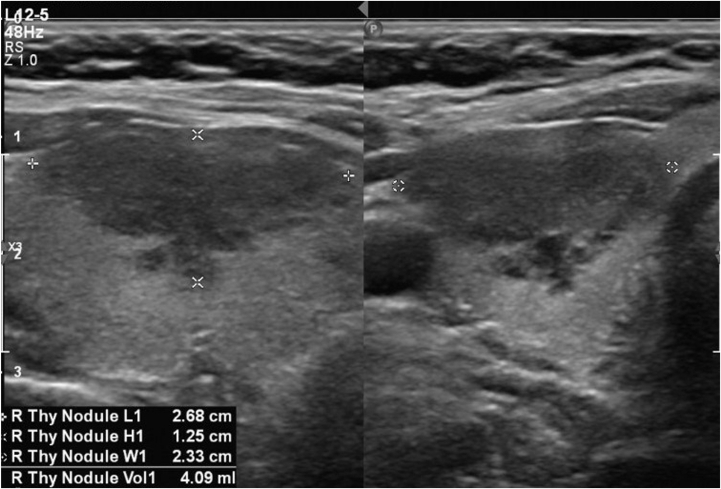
Ultrasound of thyroid demonstrating a 2.7 cm right-sided hypoechoic nodule with irregular margins.
